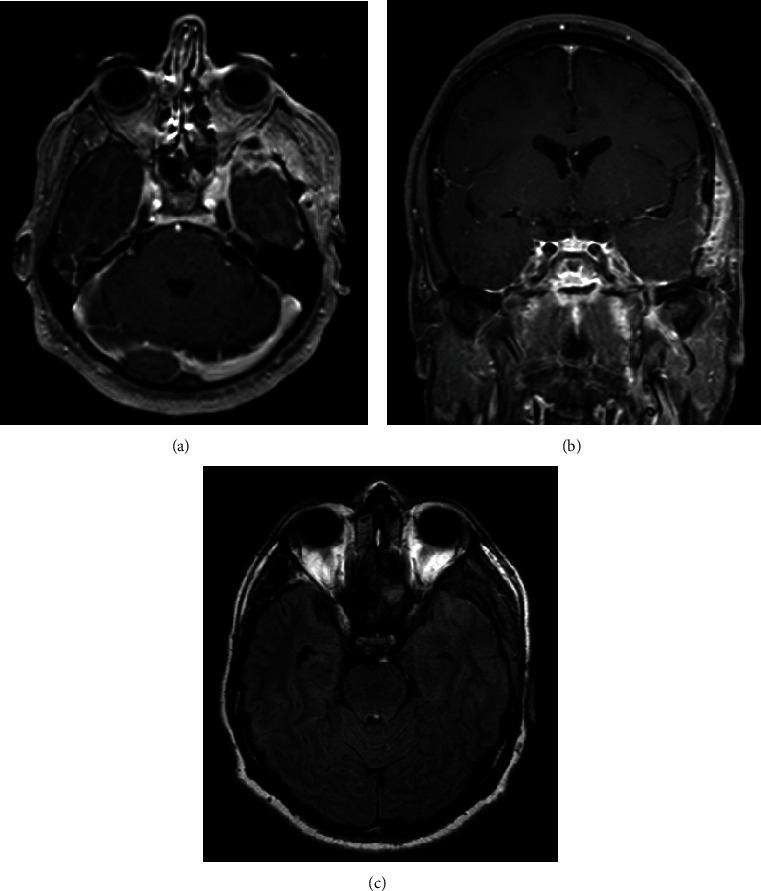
(a) Axial T1-weighted brain MRI with contrast. (b) Coronal T1-weighted brain MRI with contrast. (c) Axial T2-weight fluid-attenuated inversion recovery brain MRI. (a, b) Follow-up images after 9 months of resection demonstrating persistent pachymeningeal enhancement in the left temporal lobe with no evidence abscess formation. (c) There is resolution of the vasogenic edema in the left temporal lobe.
